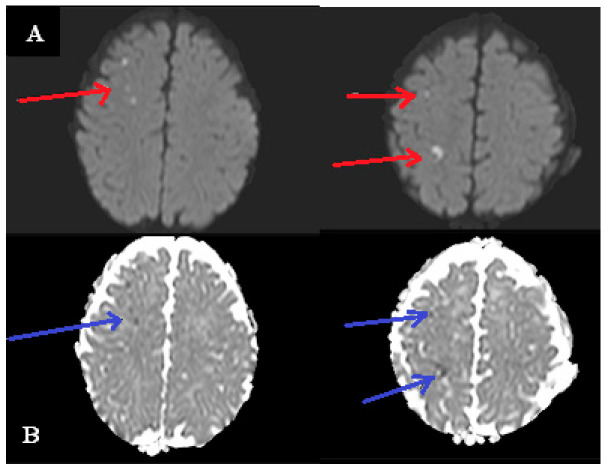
There are small foci of hyperintense signal (red arrows) on DWI (A) with corresponding hypointense signal (blue arrows) on the ADC map (B) within the white matter of the right cerebral hemisphere, seen at different axial levels. These findings are consistent with areas of restricted diffusion, in keeping with acute infarction.
